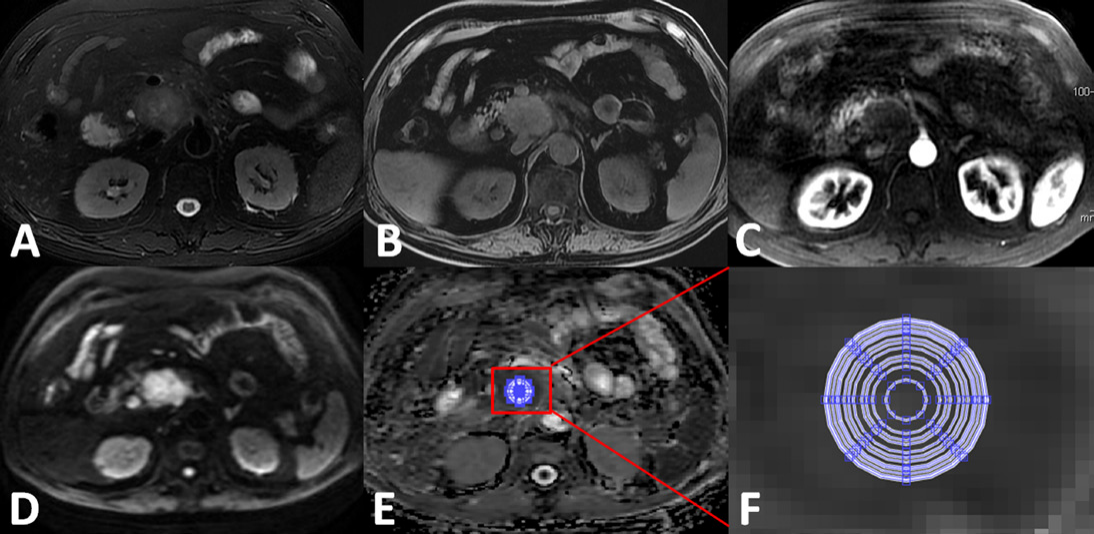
Apparent diffusion coefficient (ADC) measurements for pancreatic ductal adenocarcinoma at the head of the pancreas (A) Axial T2WI; (B) Axial precontrast T1WI; (C) Axial contrast-enhanced arterial phase T1WI demonstrating the hypovascularity of the mass; (D) DWI image (b = 600 s/mm^2^) clearly demarcated hyperintensity while compared with the surrounding pancreas tissues; (E) ADC map; (F) zoomed-in ADC map that indicates 12 concentric round ROIs (areas 15, 46, 55, 82, 99, 121, 134, 161, 189, 214, 223, and 245 mm^2^ were used for mean ADC measurements.
